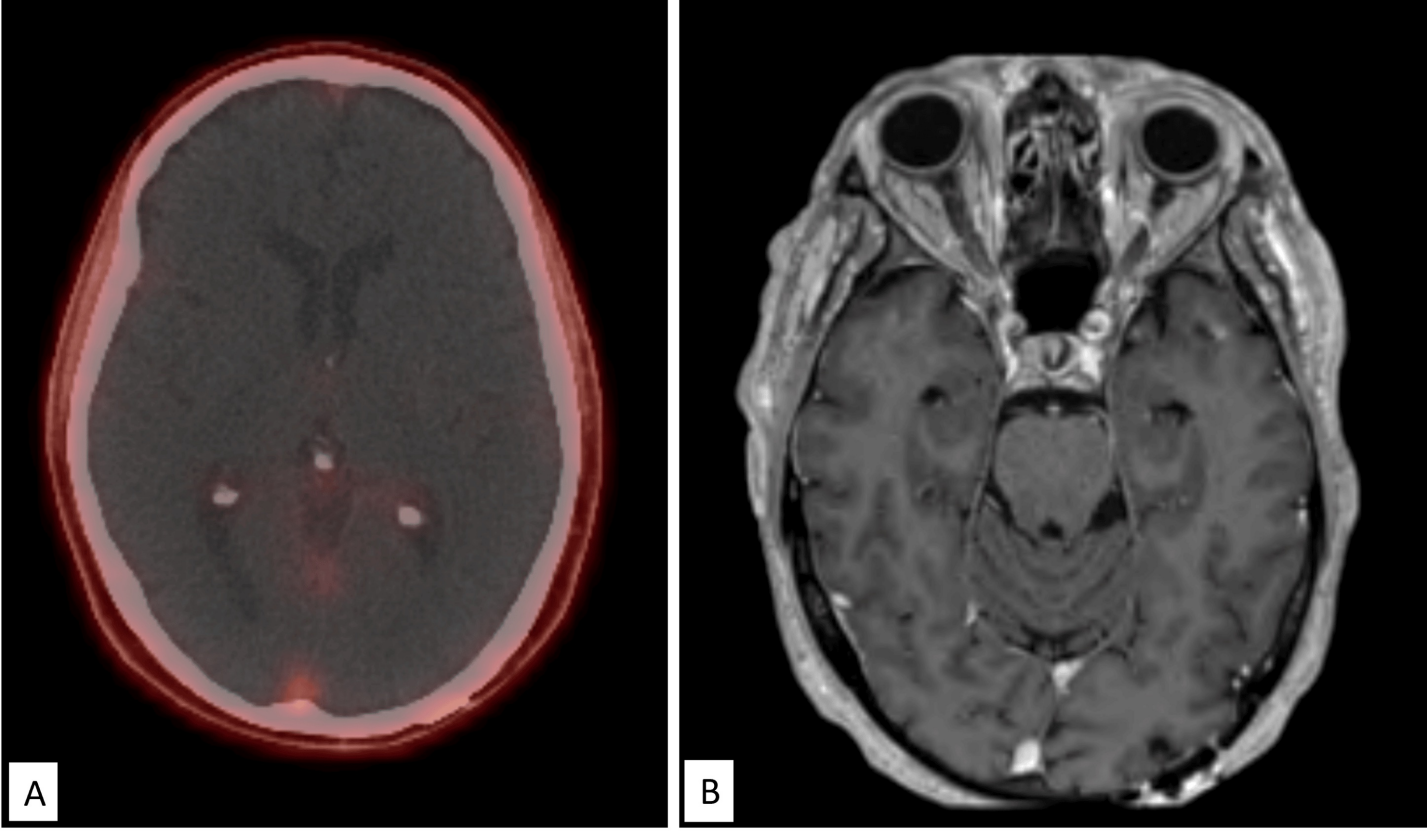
Posttreatment imaging at 15 months following GKRS (A) Complete metabolic resolution of focal PSMA activity in the left occipital lobe peripherally. (B) High-resolution gadolinium double-contrast MRI of the brain showing posttreatment encephalomalacia but no evidence of recurrent or residual malignant disease. GKRS, Gamma Knife radiosurgery; PSMA, prostate-specific membrane antigen
